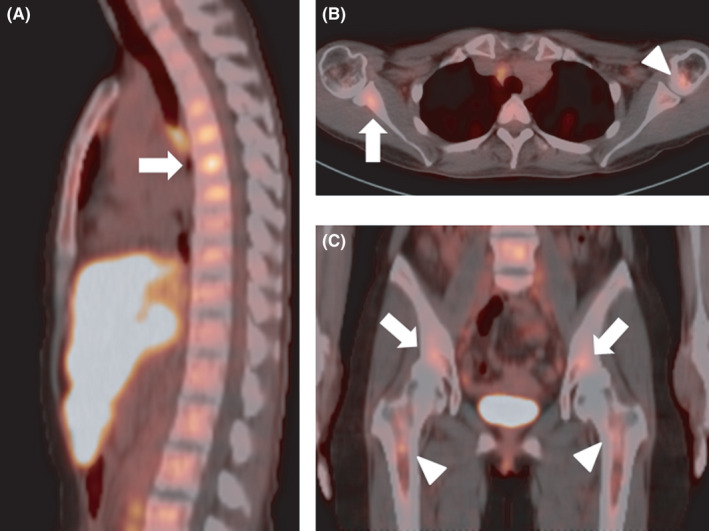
(A) Sagittal FDG PET/CT shows FDG uptake in the 4–7th thoracic vertebrae (arrow). (B) Axial FDG PET/CT shows FDG uptake in the scapula (arrow) and humerus (arrowhead). (C) Coronal FDG PET/CT shows FDG uptake in the pelvis (arrows) and femur (arrowheads)
